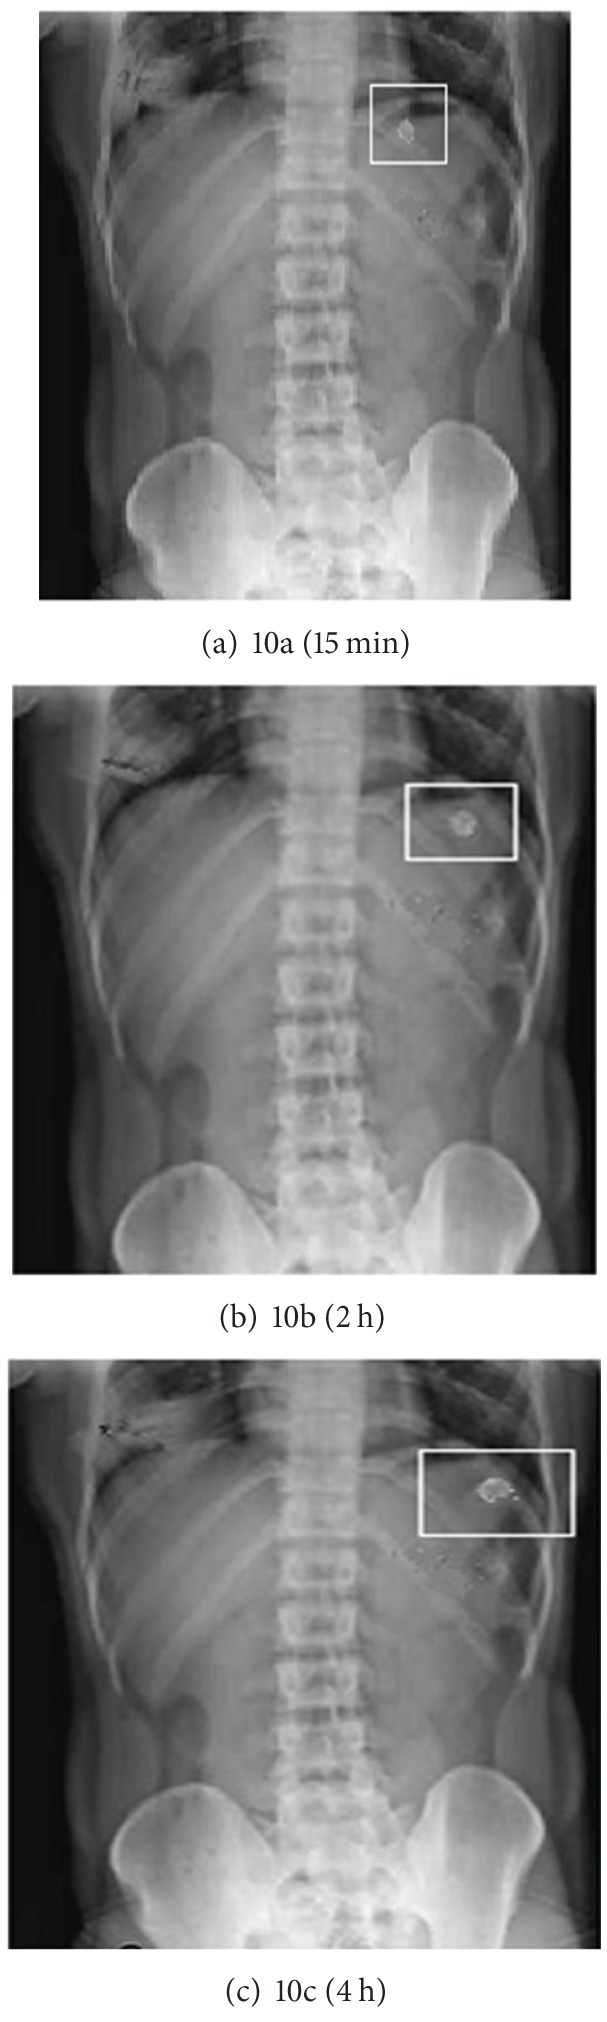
X-ray of formulation F13 at different time intervals.
